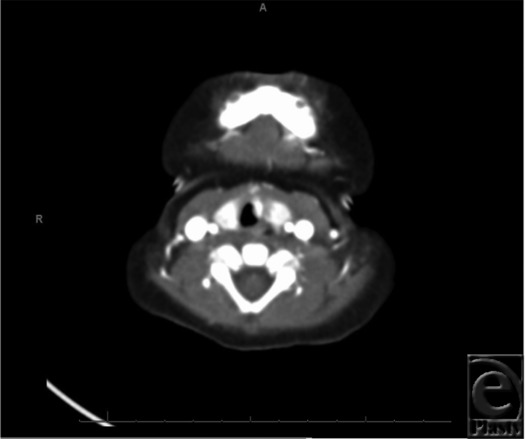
Pretreatment CTA of the neck.
